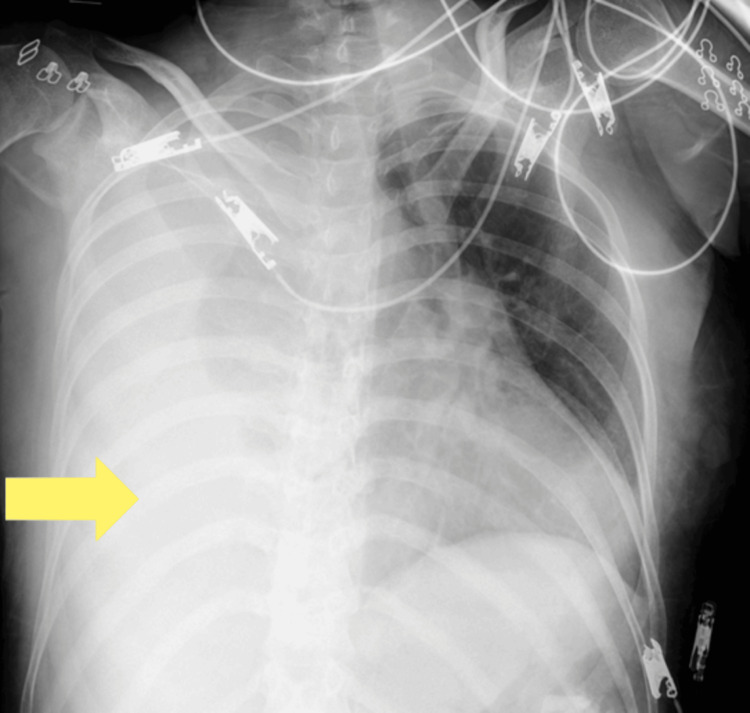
Chest radiograph Chest radiograph showing mediastinal shift and right atelectasis.
